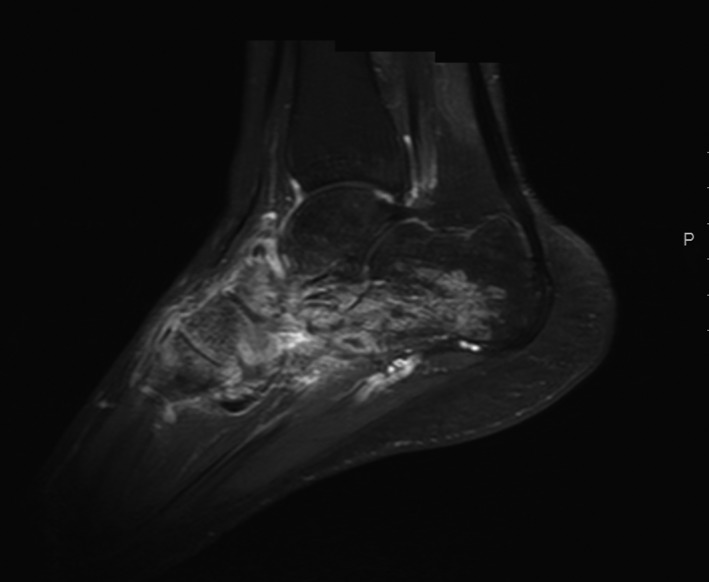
Imaginary characteristics in foot MRI indicated the presence of arthritis with disorganization of tarsal joints.
